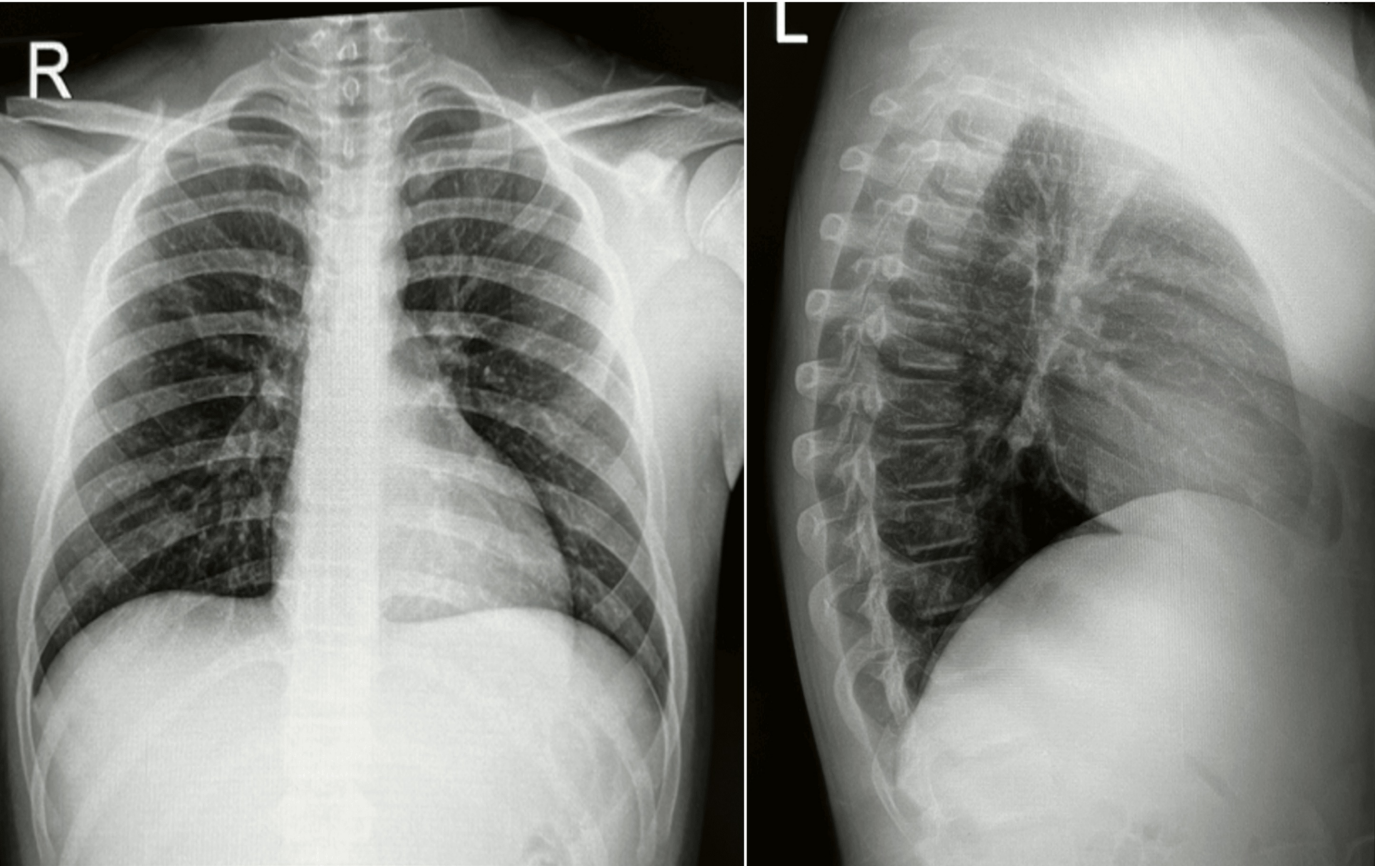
Normal Chest X-ray (Anteroposterior and Lateral Views) Showing No Acute Pulmonary Findings
